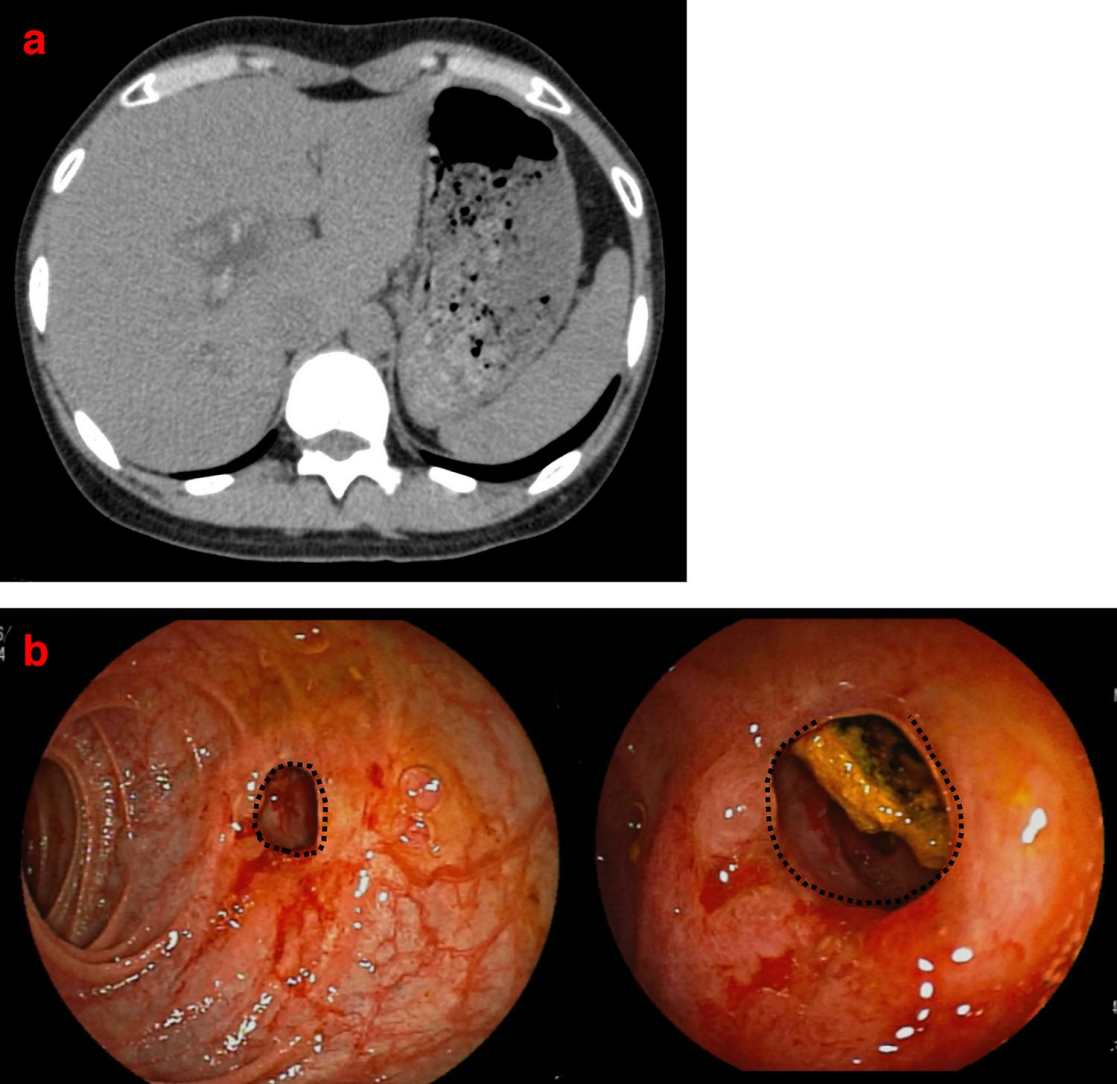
Case 3 from Table 4. (a) Stones are identified on computed tomography twelve years after radical surgery. (b) An anastomotic stricture was identified using DBERC. (dotted line) Balloon dilation and lithectomy were performed. *DBERC* double-balloon endoscopic retrograde cholangiography.
